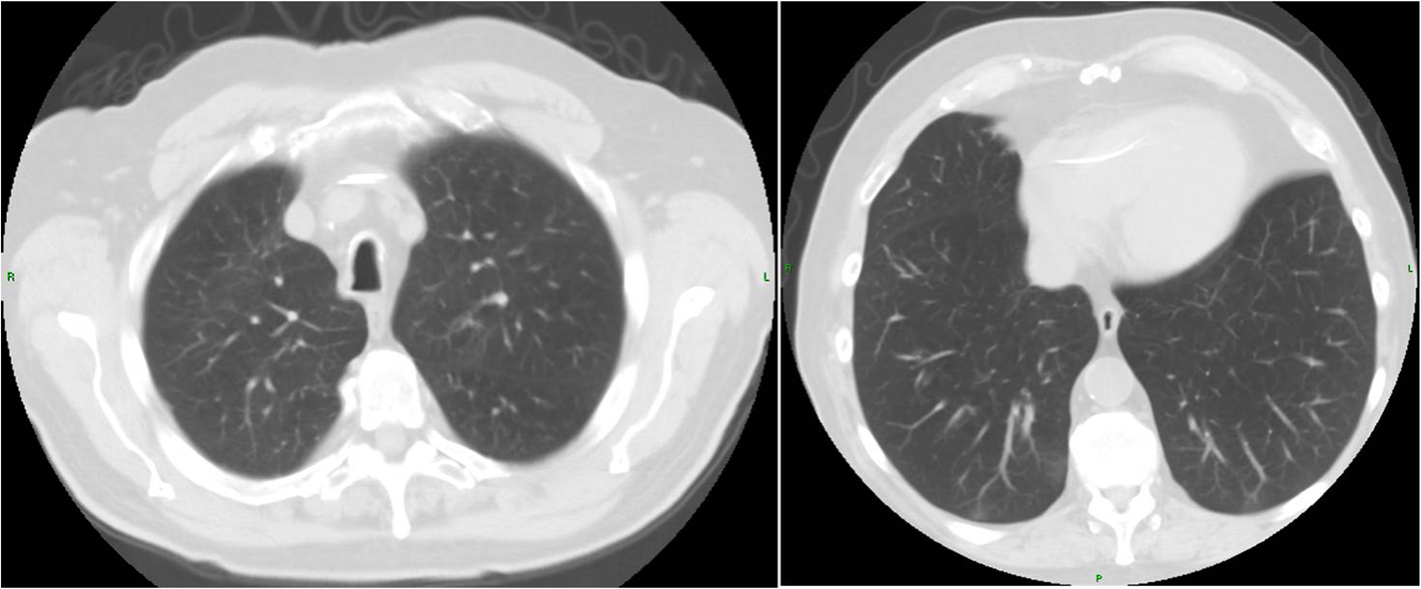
HRCT thorax of the PiFF homozygote. There is no radiological evidence of emphysema or bronchiectasis.
